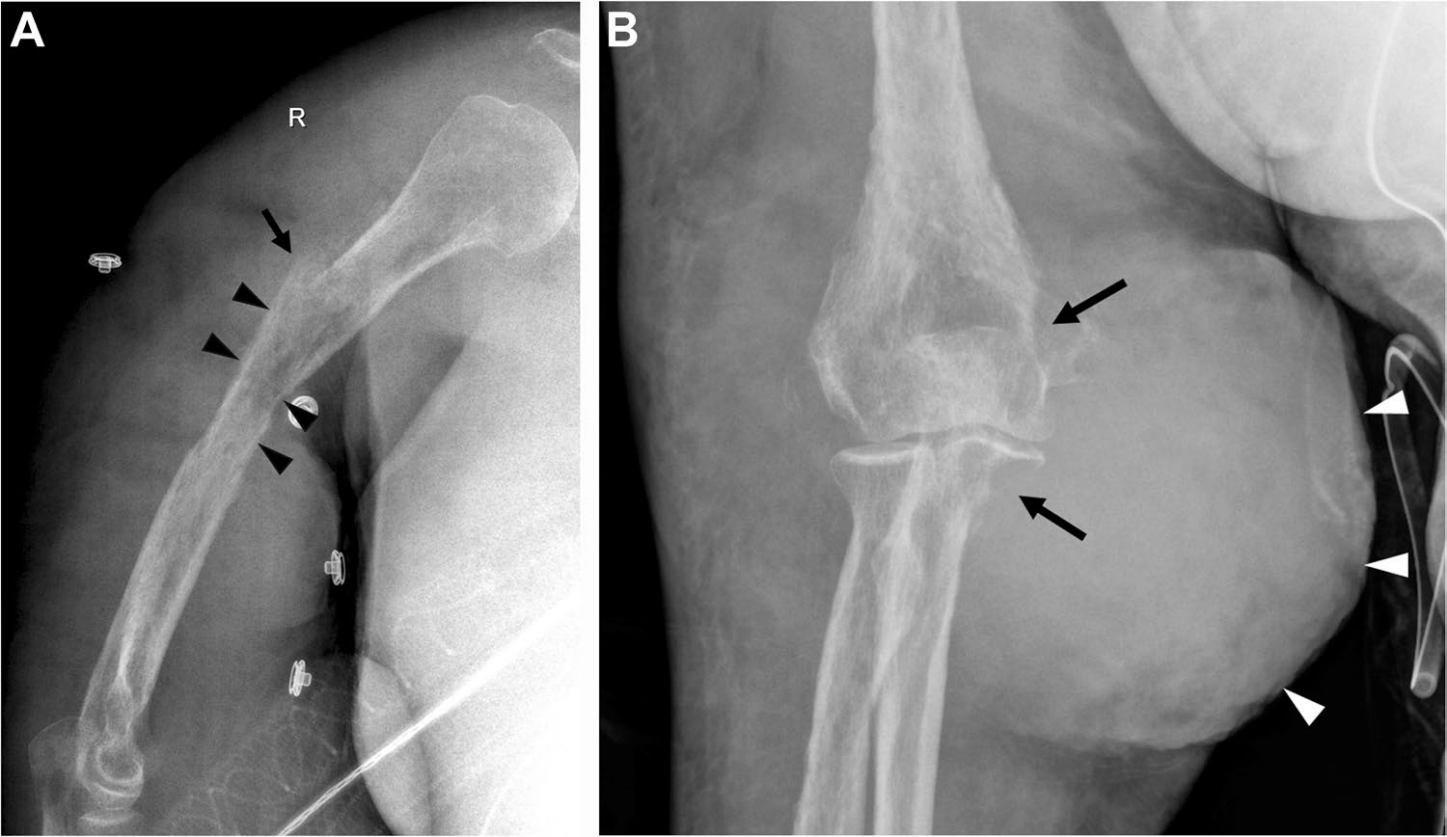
Lateral radiograph of the right humerus (A) demonstrates an aggressive permeative lesion of the right humerus (black arrowheads) with a pathological fracture (black arrow). AP radiograph of the elbow (B) shows aggressive lesions of the distal humerus and proximal ulna (black arrows) and large soft tissue mass medial to the elbow (white arrowheads)
